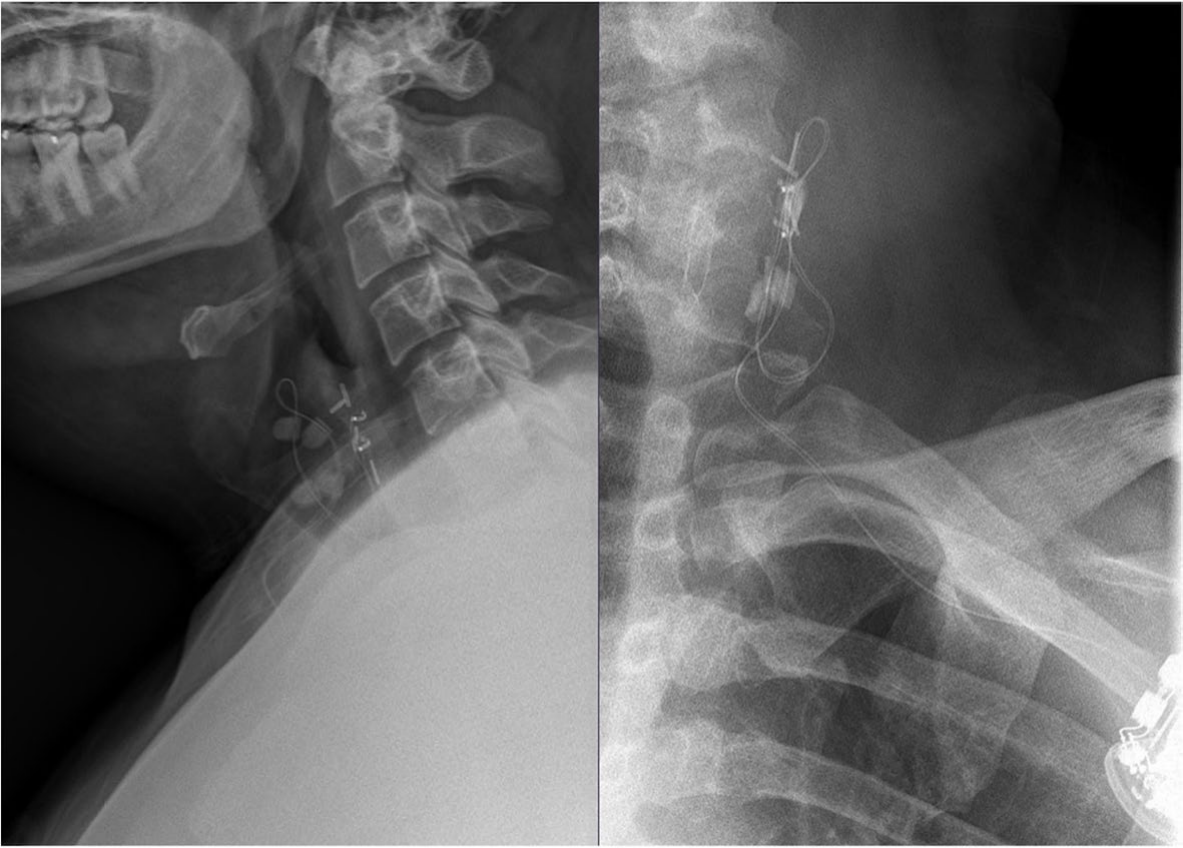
Preoperative plain radiograph of the neck demonstrating VNS electrode helical contacts at the C5 level and no visible VNS hardware discontinuity
